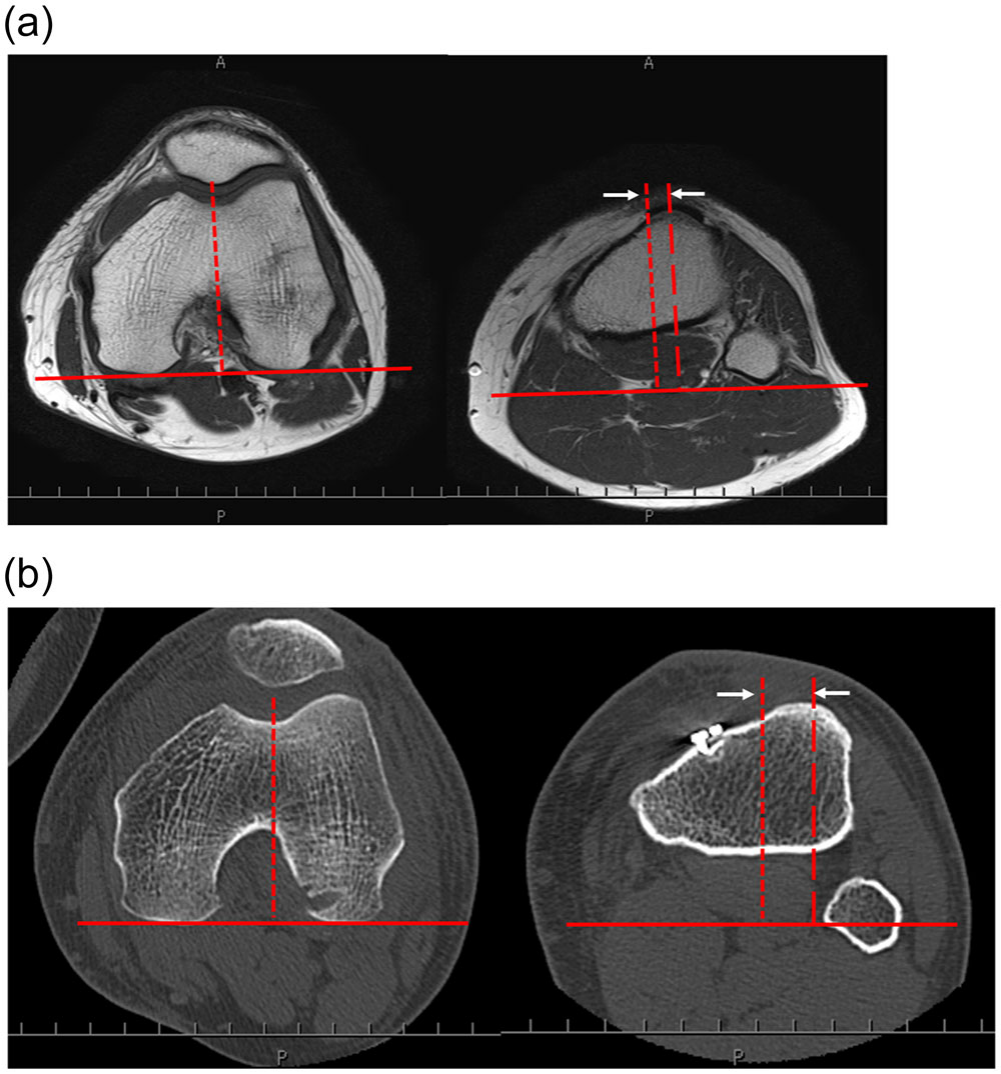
Measurement of tibial tubercle‐trochlear groove (TT‐TG) distance on magnetic resonance imaging (MRI) and computed tomography (CT). The TT‐TG distance is assessed using axial sequences. It is defined as the offset distance between the deepest point of the trochlear groove and the centre of the patellar tendon insertion on the tibial tuberosity. The most posterior point of the medial and lateral condyles of the femur is defined as the reference line (solid line). A line perpendicular to the reference line is drawn through the deepest bony point of the trochlear groove (dotted line). A second line perpendicular to the reference line is drawn through the most anterior point of the tibial tuberosity on the axial section, showing the most anterior point of the tuberosity (dashed line). The TT‐TG distance is defined as the distance between these two perpendicular lines (arrow). A positive value indicates external rotation of the tibia relative to the femur. (a) Measurement of TT‐TG distance on MRI. (b) Measurement of TT‐TG distance on CT.
